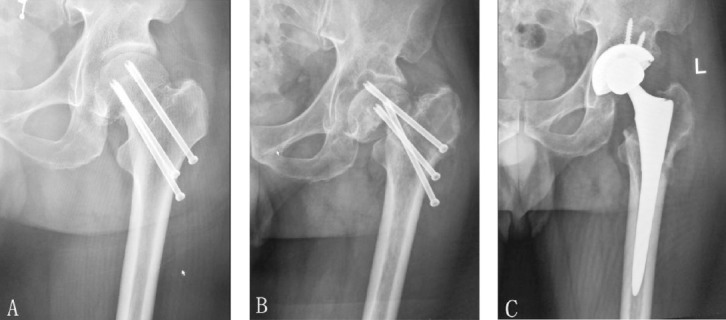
Anteroposterior radiograph of a 68-year-old male showing femoral neck fracture treated with cannulated screws fixation (A). Nonunion result in cut-out of the internal device at one year after operation (B). Anteroposterior radiograph 2 year after salvage uncemented total hip arthroplasty showing good prosthesis position (C).
